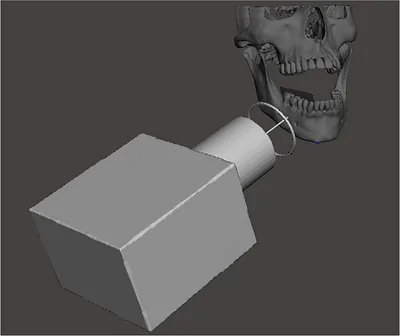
Radiographic technique during device development in Meshmixer.
